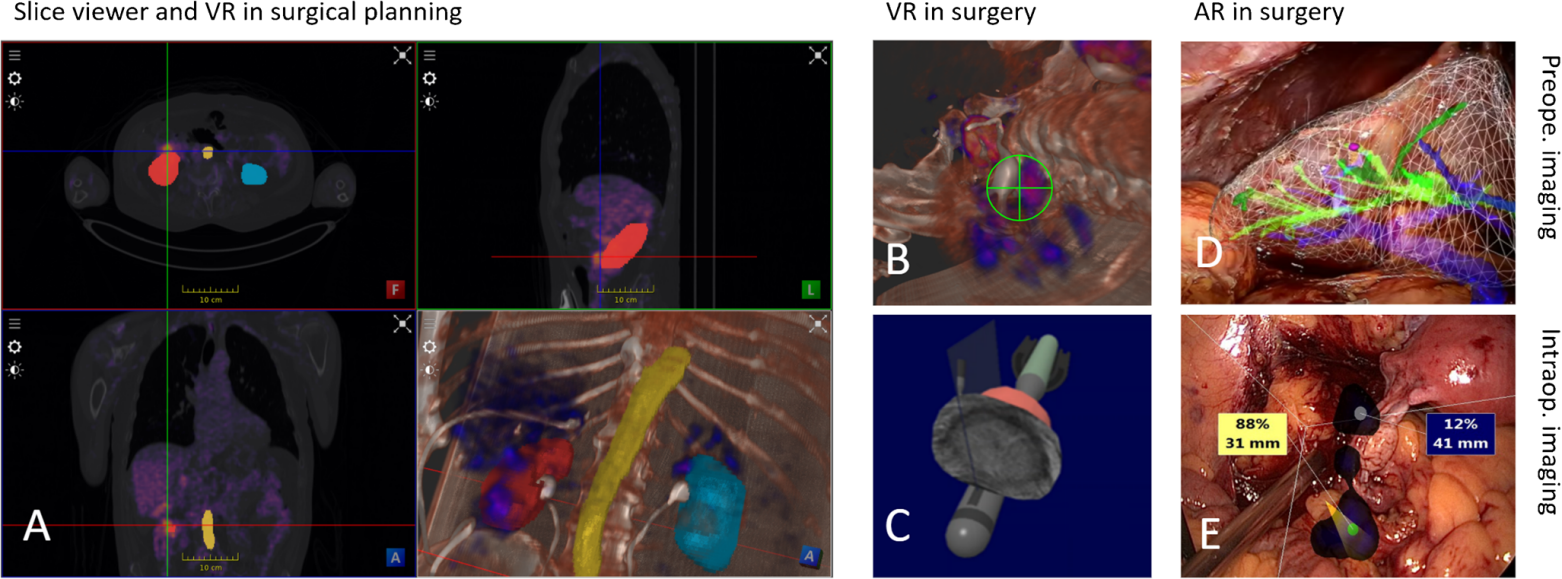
Different VR/AR visualization options applicable for robotic surgery. A Visualization of PET/CT in 3 planes (axial, coronal, and sagittal) and 3D render, including an overlay of segmented organs. B VR view of PET/CT image as used for guidance on PSMA-guided surgery. C VR view of intraoperative TRUS in the context of the TRUS probe and the robot instruments. Image courtesy of Tim Salcudean, UBC, Canada. D Segmented organs overlaid as AR patients’ body for port placement planning [80]. E AR visualization of freehand SPECT images showing sentinel lymph nodes in an endometrium cancer surgery
